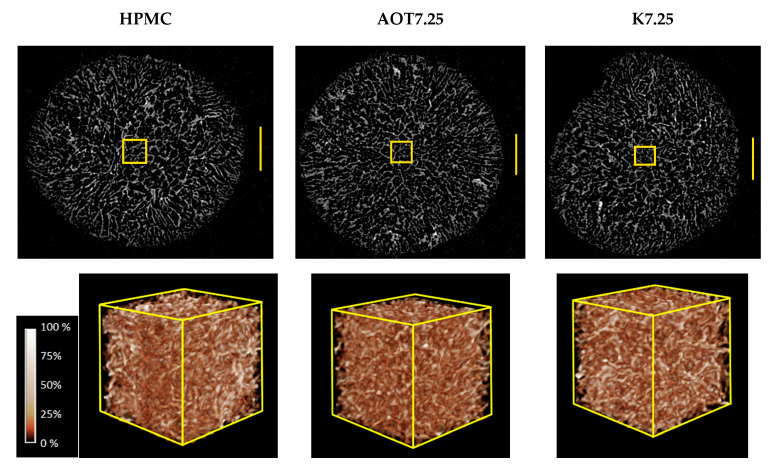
Reconstructed 3D models from microtomography data of HPMC, AOT7.25, and K7.25 cryogel samples. The top images correspond to transversal slices of the total diameter (ø = 5 mm), and the scale bale corresponds to 1 mm. The cubes (500 µm × 500 µm × 500 µm) correspond to the central region of the samples; the attenuation scale was obtained using the transfer function in the software CTVox^®^. Black and white correspond to the minimal and maximal X-ray attenuation, respectively.
